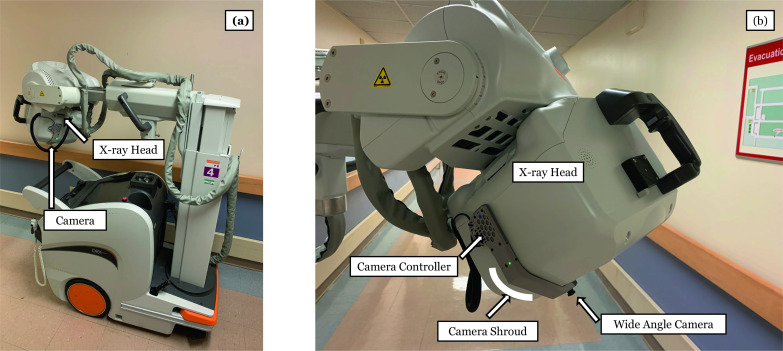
a Point-of-care camera attached externally to a portable radiography machine. b Close-up view of the camera, camera controller, and camera shroud on the X-ray head of a portable radiography machine
